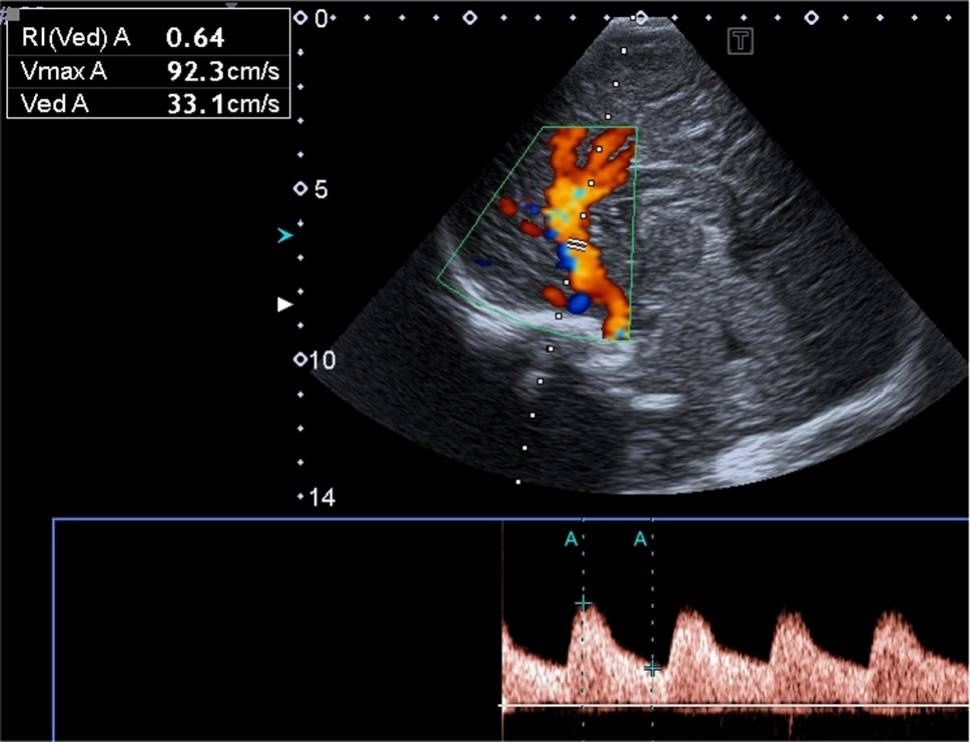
Doppler flow pattern of the major arteries show normal velocities
